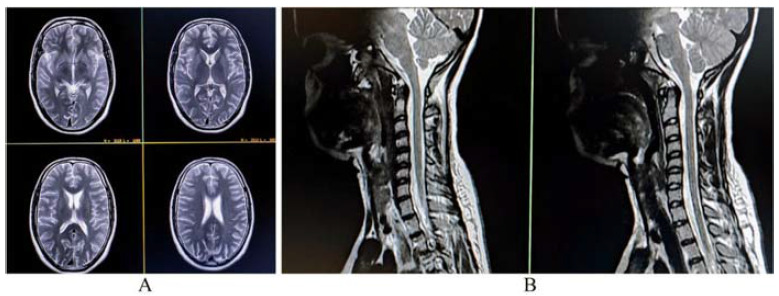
Magnetic resonance imaging of the brain revealing no abnormalities on axial tbl2-weighted imaging (A). Sagittal tbl2-weighted imaging of the cervical spine also was normal (B).
